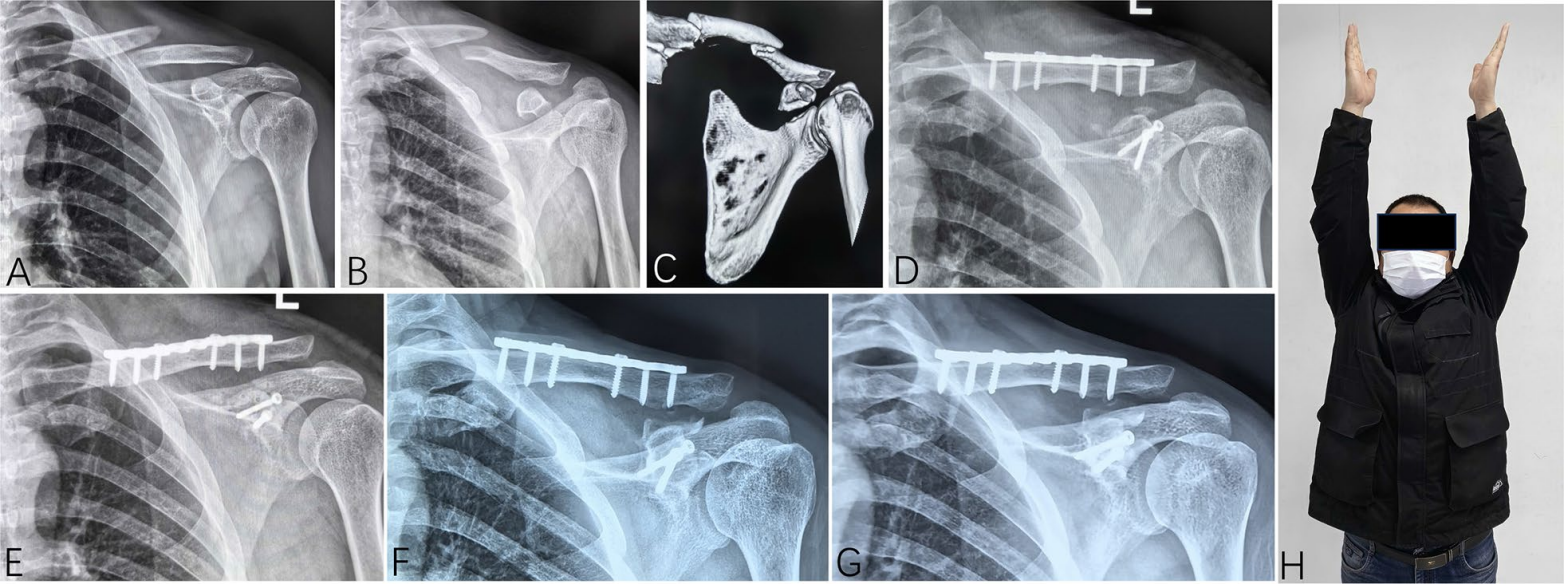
A 43-year-old man (patient 23) sustained a displaced clavicle shaft fracture and a displaced ipsilateral Eyres type III coracoid process fracture due to a fall from height. A–C Preoperative imaging showed the fracture. D, E He was treated with open reduction and internal fixation. F, G X-rays taken 12 months postoperatively showed solid bone union with no implant failure. H He achieved a full range of shoulder motion and returned to his pre-injury work
